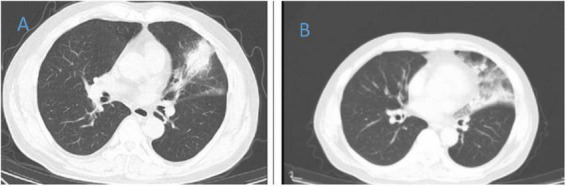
Chest CT of patient 3. (A) Before treatment on 2021.12.11; (B) the follow-up imaging 1 week after treatment showed progression compared to the pre-treatment scans.
